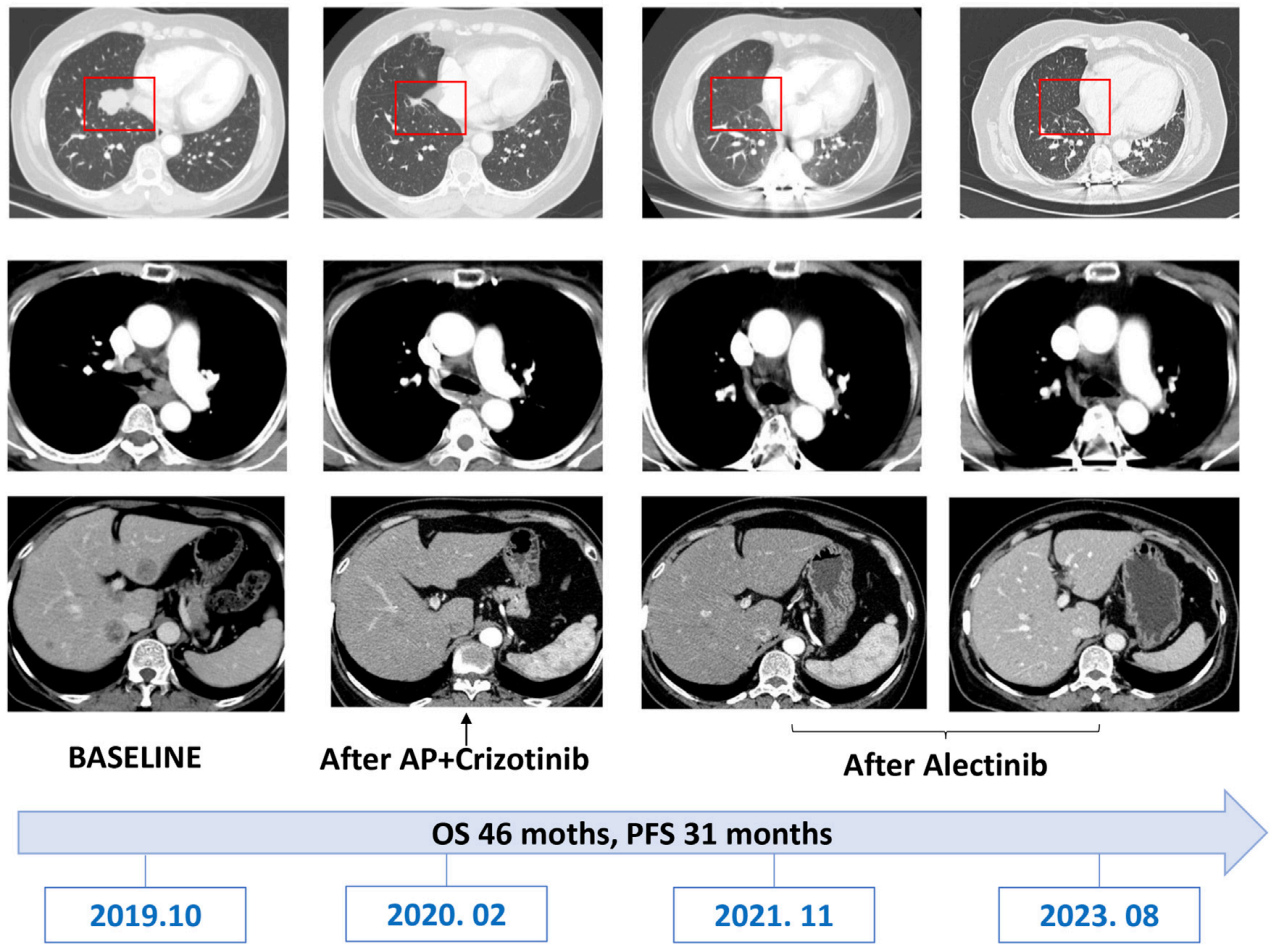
CT Imaging of the reported patients with follow-up.
